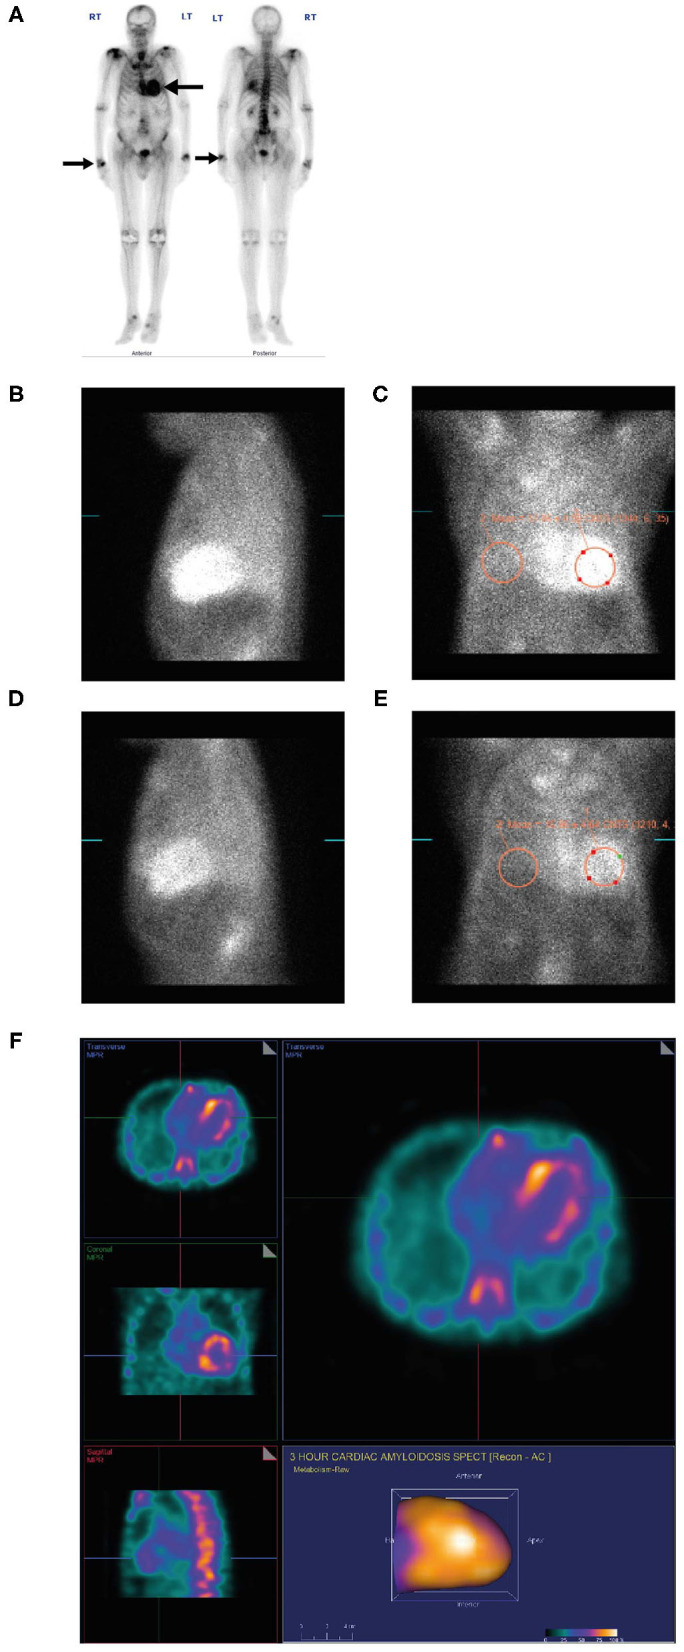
PYP scan (A) for metastatic prostate cancer showing an incidental finding of intense myocardial uptake of bone tracer (Grade 3, black arrow), greater than the ribs and spine and intense uptake in both wrists (black arrow) consistent with diagnosis of carpal tunnel syndrome. Lateral planar (B) and anterior planar (C) scans demonstrate intense cardiac PYP uptake at 1 h with H/CL ratio 1.67. At 3 h, lateral planar (D) and anterior planar (E) scans demonstrate intense cardiac PYP uptake with H/CL ratio 1.77. SPECT scan (F) demonstrates intense myocardial uptake in the RV and LV free walls with no blood pool in the left ventricular cavity. H/CL, heart to contralateral lung ratio; LV, left ventricular; PYP, pyrophosphate; RV, right ventricular; SPECT, single-photon emission computed tomography.
